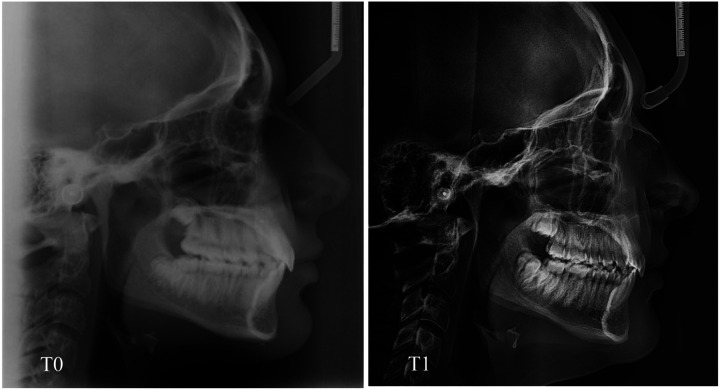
Lateral cephalometric radiography at time T0 and T1 of a patient in the AG.
